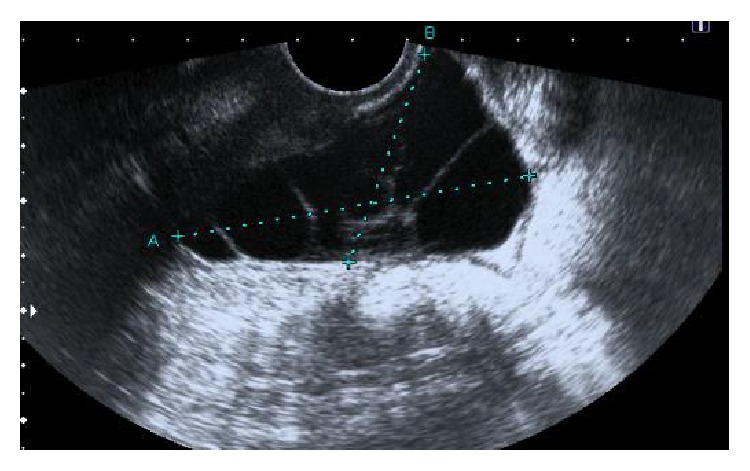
Ultrasound scan image of pelvic multicystic mass.
